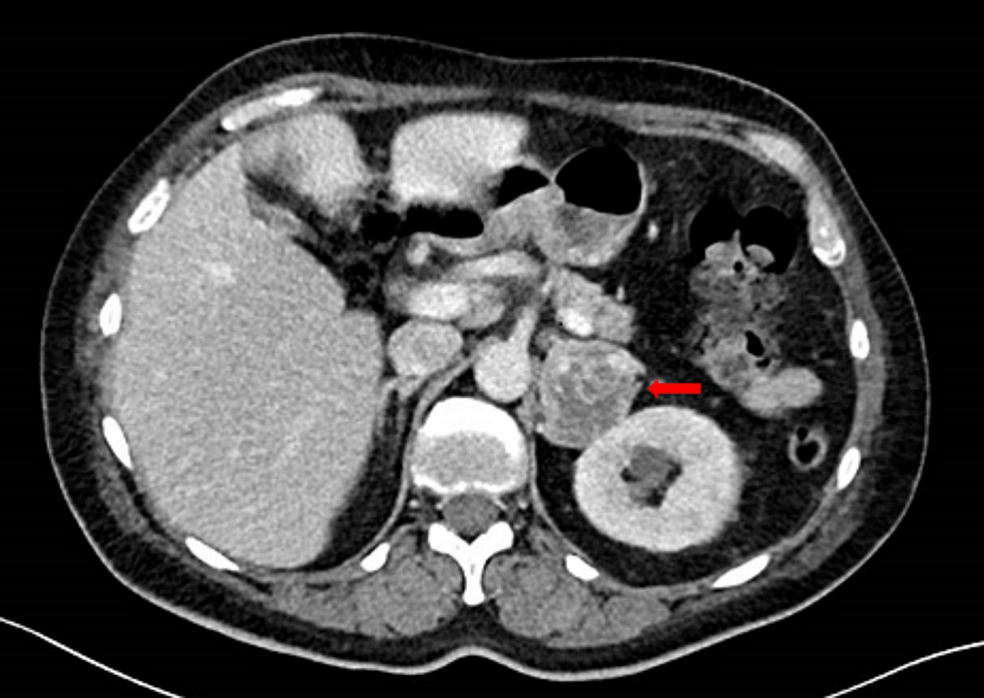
Adrenal mass noted on CT scan
